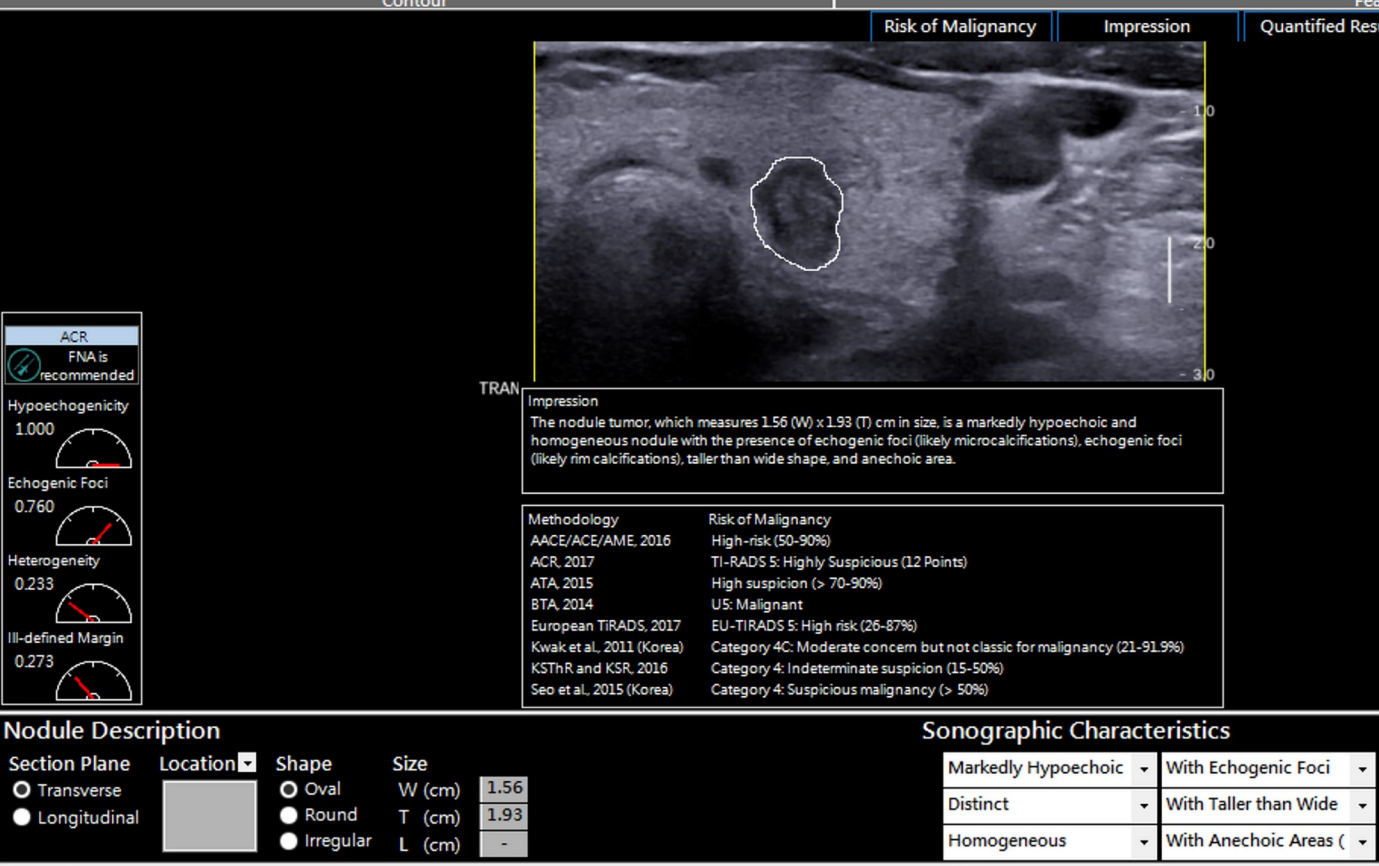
An example of a solid markedly hypoechoic thyroid nodule that was diagnosed as false positive. The nodule was also taller than wide with echogenic foci. The impression of these suspicious sonographic features led to the misdiagnosis by the different TIRADS within the AmCAD-UT software. The nodule was diagnosed as benign with cytology.
